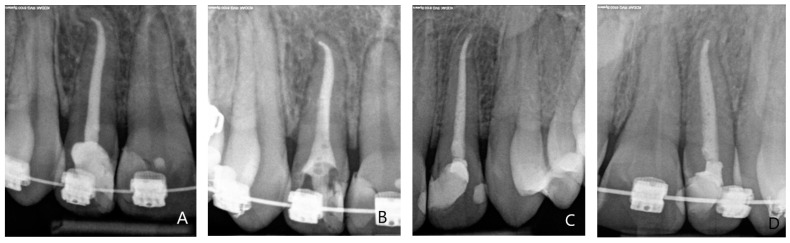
An anterior intraoral periapical radiograph taken during treatment with fixed orthodontic appliances. (A,C) Baseline intraoral periapical radiograph taken at the first appointment: Endodontically treated lateral maxillary incisor with atypical root shape (root apex curvature), maxillary central incisor (vital), with a typical morphology of the root. (B,D) Intraoral periapical radiographs taken at the third/fourth appointment: Mild external apical root resorption of lateral maxillary incisor and flattening of the apex of central maxillary incisor.
